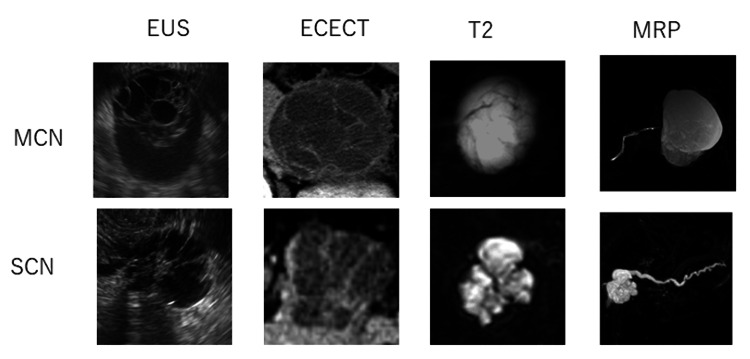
Input data for four modalities used in this study. MCN: mucinous cystic neoplasms, SCN: serous cystic neoplasms, EUS: endoscopic ultrasonography, ECECT: early phase of contrast-enhanced computed tomography, T2: T2-weighted magnetic resonance imaging, MRP: magnetic resonance pancreatography
